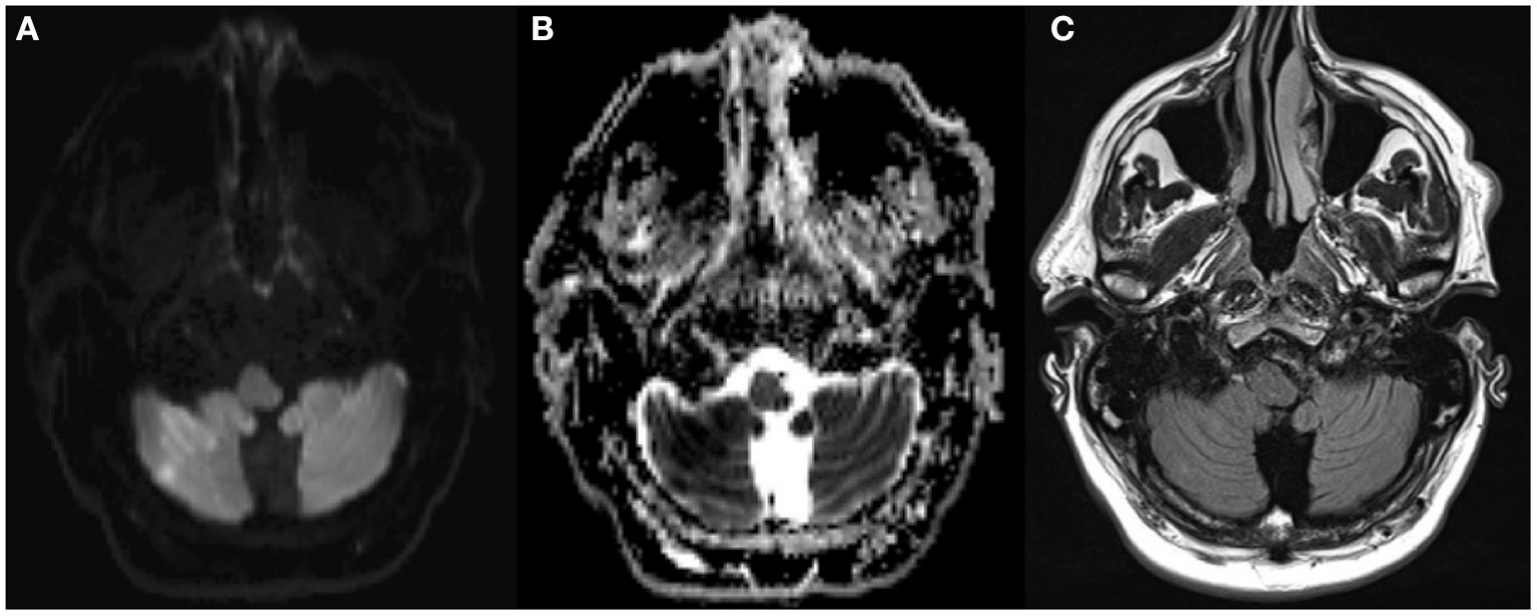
Fifty-five year old man with acute onset of mild dysathria and ataxia of the trunk, unable to walk (NIHSS 1). MRI showed an acute infarction [DWI positive (A), ADC negative (B), FLAIR negative (C)] in the territory of the occluded right posterior inferior cerebellar artery. The patient was treated with rtPA 3 h and 46 min after stroke onset.
